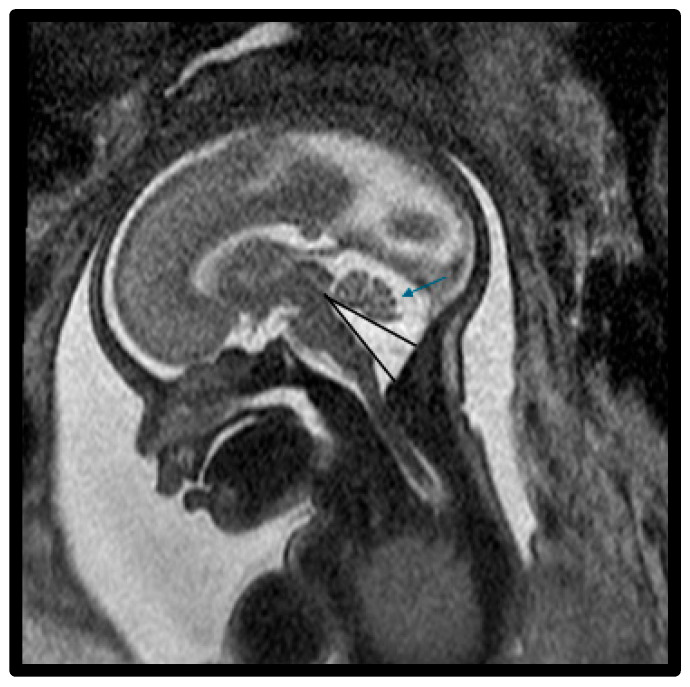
Fetal MRI image of a fetus at 25 gestational weeks with vermian hypoplasia. The midline T2-weighted sagittal image shows an enlarged fourth ventricle, decreased vermian biometry, and enlarged fluid space of the posterior fossa. There was mild hypoplasia of the postero-inferior vermis with slight upward rotation (blue arrow) and increased tegmento–vermian angle (black lines). The fastigial recess was shallow and there was mild widening of the vallecula, the midline outlet of the fourth ventricle. There was a prominent and abnormal fourth ventricle with a loss of the normal diamond shape. The fourth ventricle is shown inferiorly connecting with a prominent cisterna magna through the vallecula.
